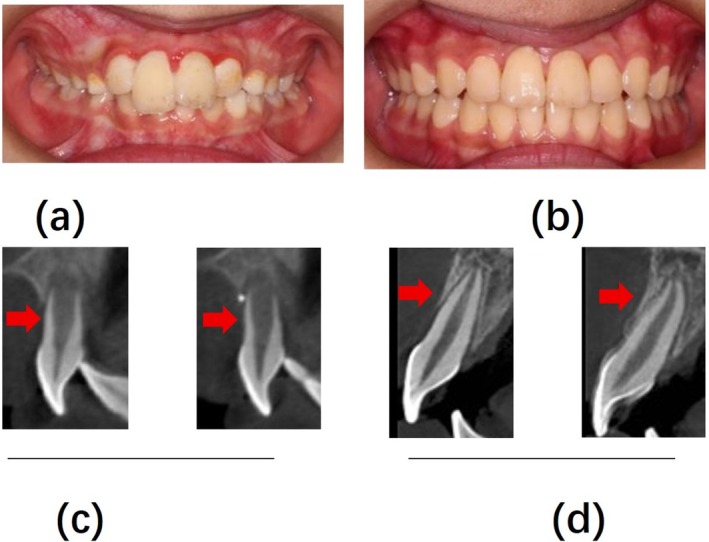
Clinical and radiograph view before and after orthodontic treatment. (a, b) Intraoral views before and after orthodontic treatment; (c–d) radiograph views before and after orthodontic treatment.
